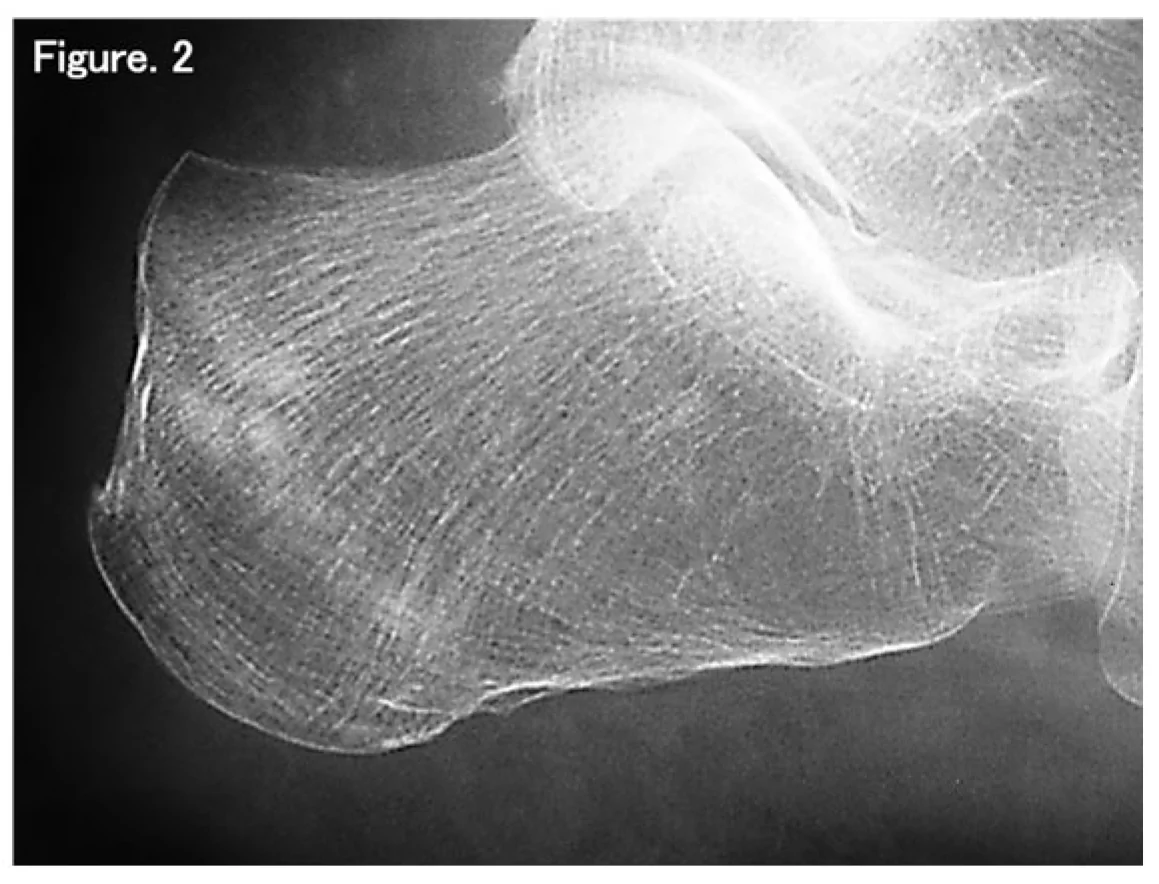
Follow-up radiograph of the same 77-year-old woman. Osteosclerotic change became apparent 2 weeks after the initial visit, supporting the diagnosis of calcaneal insufficiency fracture.
